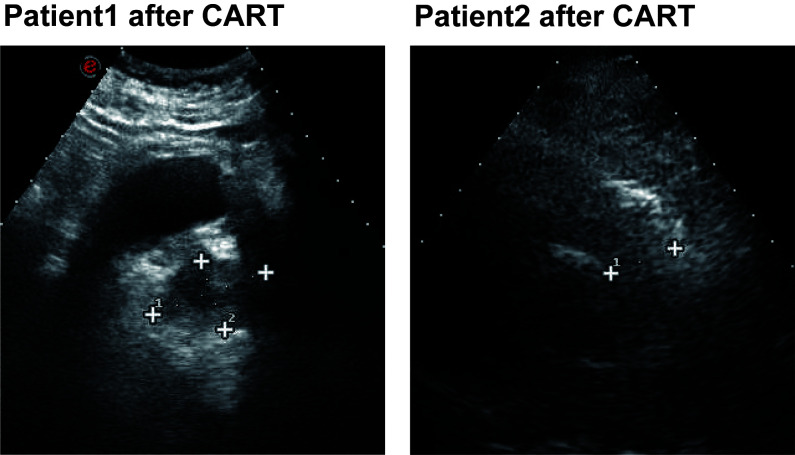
Ultrasound images of the thyroid gland after CART treatment in two patients.
